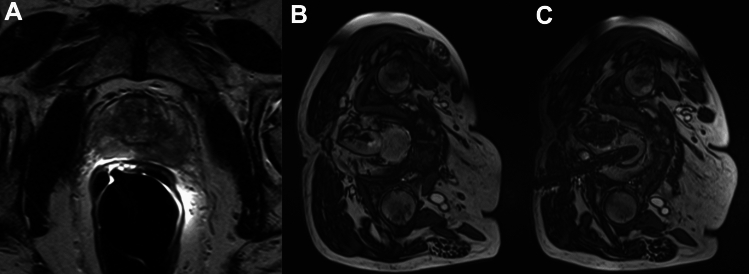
Case Presentation (72 year-old patient) In the current multiparametric MRI of the prostate, the axial T2-weighted sequence a demonstrates a left-sided, confluent, ill-defined, hypointense lesion located in the peripheral zone at the level of the prostatic base, suspicious for prostate cancer. For targeted detection and biopsy planning, image b shows the planning scan used for biopsy. During tissue sampling, the MR guidance image c confirms the correct positioning of the biopsy needle within the left-sided index lesion
